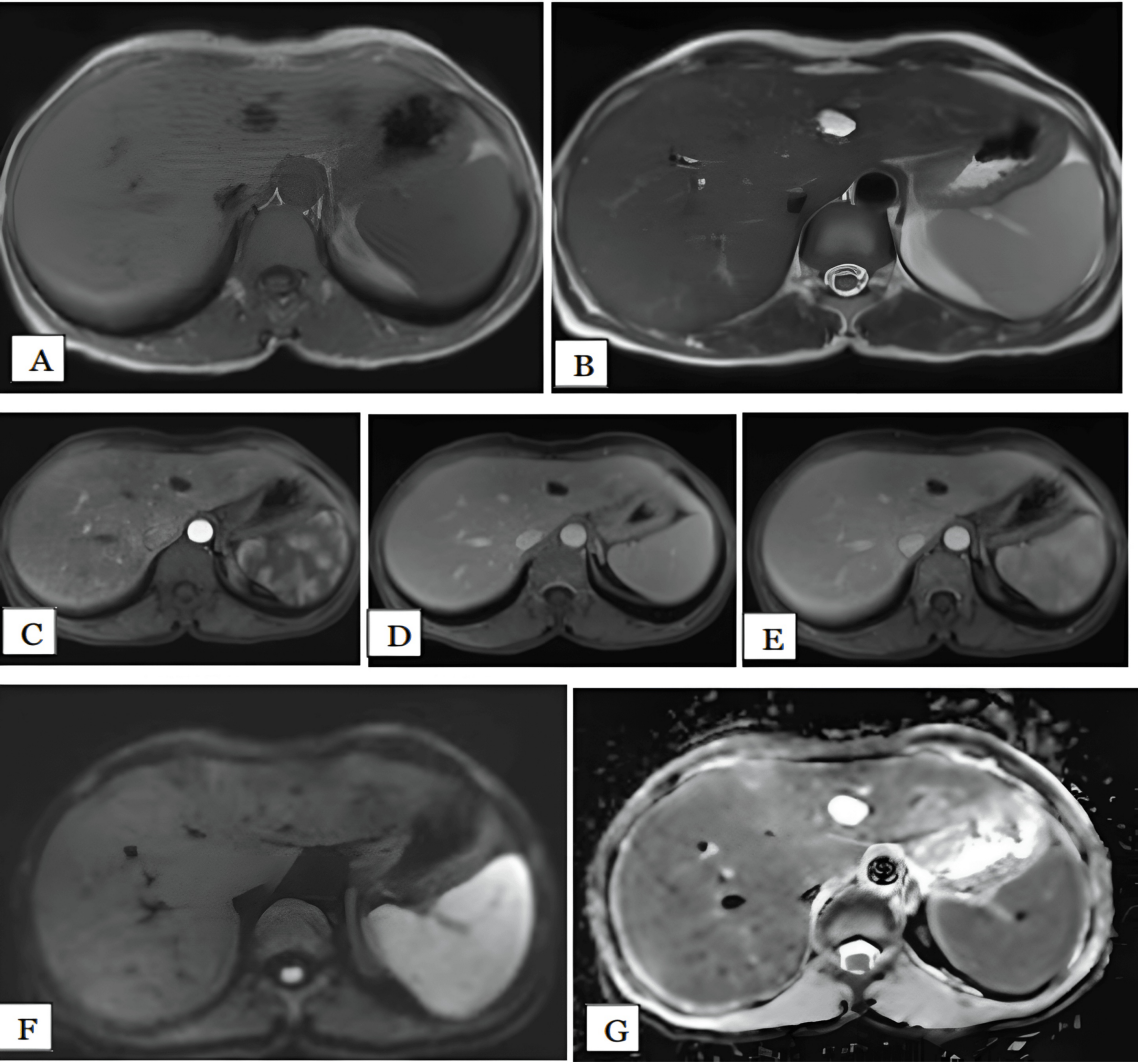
Simple hepatic cyst in a 44-year-old female Axial T1 (A), T2 (B), arterial (C), portal, (D), and delayed (E) phase MRI images show well-defined T1 homogenous hypointense. T2 homogenous hyperintense lesion was noted in segment IVa of left lobe of liver showing no enhancement post contrast. DWI (F) and ADC (G) maps show the lesion is isointense on DWI with hyperintense on ADC maps with mean ADC value of 3.2 x 10^-3 ^mm^2^/s, suggestive of facilitated diffusion. DWI: Diffusion-weighted imaging; ADC: Apparent diffusion coefficient
